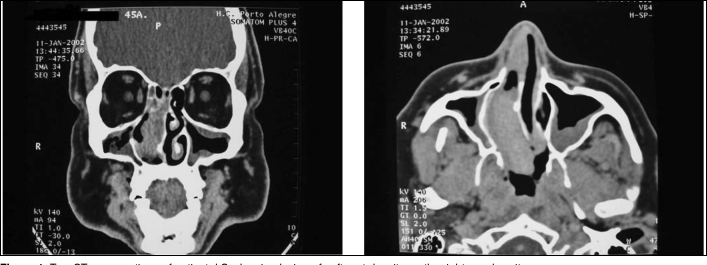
Two CT scan sections of patient J.C. showing lesion of soft part density on the right nasal cavity.
